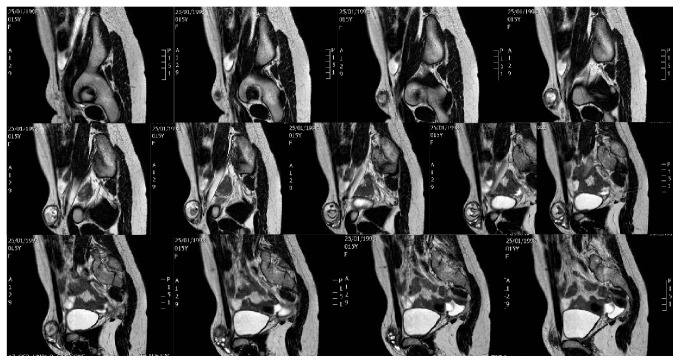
MRI study revealing the ovarian mass herniated in the inguinal region, sagittal section.
